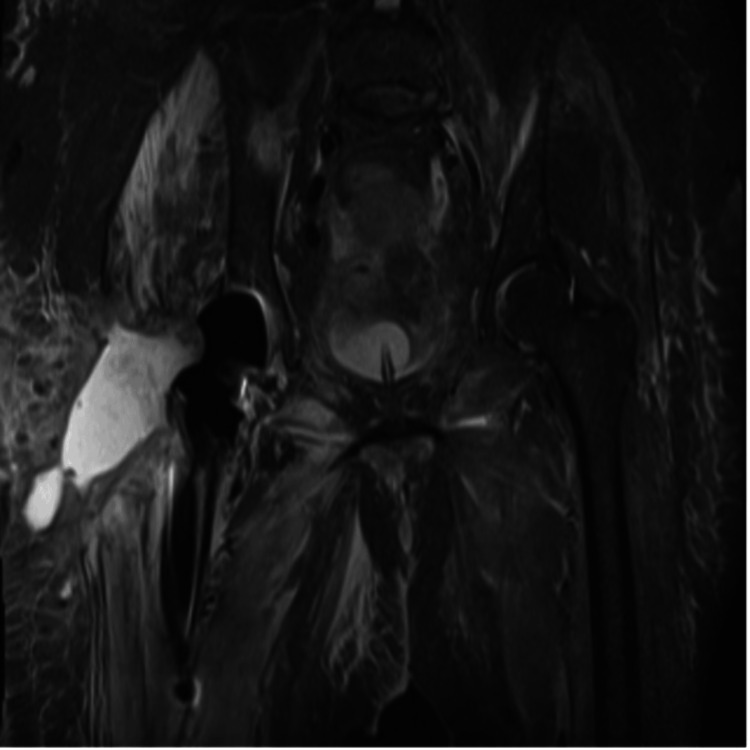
MRI during spacer phase NRI: magnetic resonance imaging
